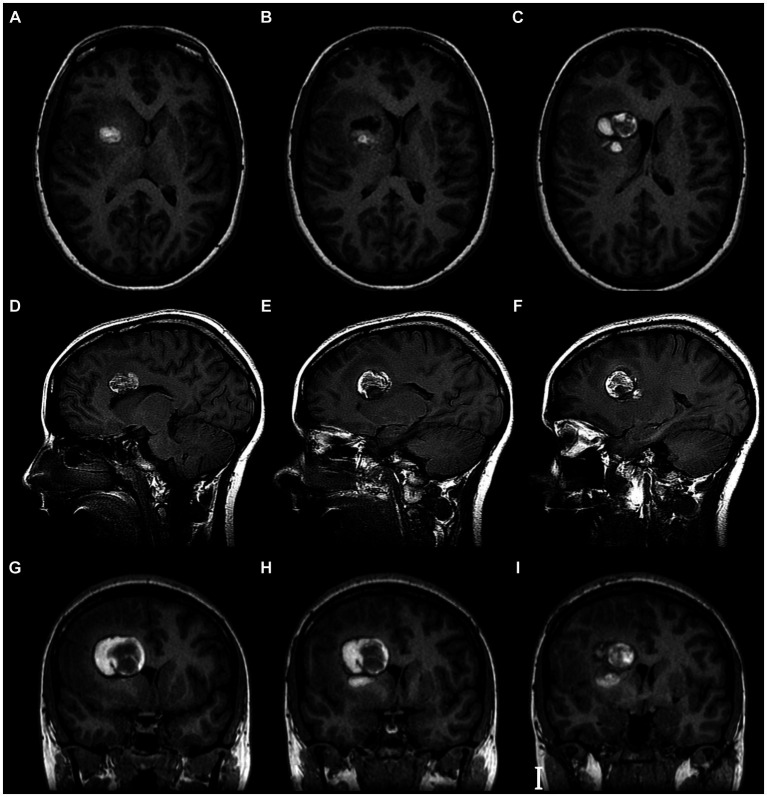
Brain MRI. T1 with Contrast of cavernoma of the anterior limb of the internal capsule. (A–C) Axial view. (D–F) Sagittal view. (G–I) Coronal view. Evidence of hypertensity image, heterogeneous, oval, with circumferential peripheral enhancement. It is intimately related to the anterior limb of the internal capsule, the head of the caudate nucleus, and the anterior horn of the right lateral ventricle.
